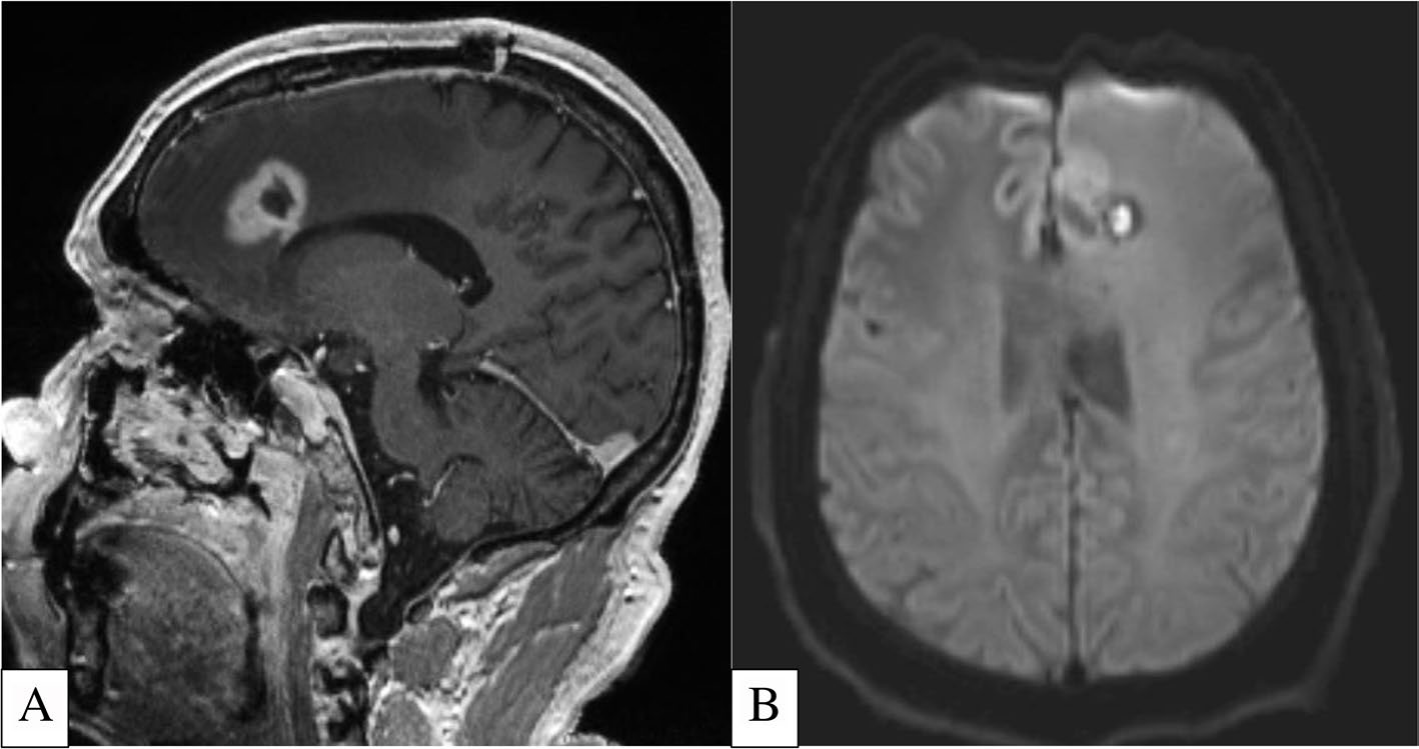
A-B 53-Year-Old Patient with Biopsy Proven Left Frontal Lobe Glioma with TERT Promoter Mutation. Sagittal T1-Weighted Post Contrast MRI (A) shows an enhancing lesion with central non-enhancing component that exhibits elevated DWI signal (B), in keeping with necrosis
